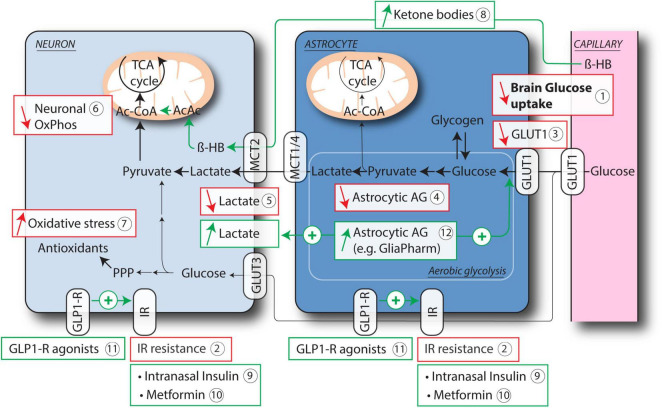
Age-related astrocytic and neuronal deficits leading to brain hypometabolism and current therapeutic strategies. Brain glucose hypometabolism is a hallmark of aging and neurodegeneration, as shown in particular by FDG PET studies (1). Resistance to Insulin has been proposed to account, at least in part, for this hypometabolism (2). Astrocytes and neurons both express insulin receptor (IR). Other key features of brain hypometabolism include reduced expression of GLUT1 on astrocytes and endothelial cells (3), decreased aerobic glycolysis (AG) in astrocytes (4) and consequent impaired release of lactate (5), reduced mitochondrial activity in neurons (6) and increased oxidative stress (7). Therapeutic strategies that aim at restoring brain energy metabolism include the use of ketone bodies as alternative energy source for neuronal mitochondrial OxPhos, either through ketogenic diet or medium chain triglycerides (8), targeting IR resistance either directly with intranasal Insulin (9) or Metformin (10), or via activation of GLP-1R (11). Another specific therapeutic approach consists in improving astrocytic AG (12), which results in increased glucose uptake and lactate release by astrocytes. Ac-CoA, acetyl-CoA; AcAc, acetoacetate; β-HB, β-hydroxybutyrate; GLP1-R, glucagon-like peptide 1 receptor; GLUT1, 3, glucose transporter 1, 3; IR, insulin receptor; MCT1, 2, 4, monocarboxylate transporter 1, 2, 4; OxPhos, oxidative phosphorylation; PPP, pentose phosphate pathway; TCA cycle, tricarboxylic acid cycle.
